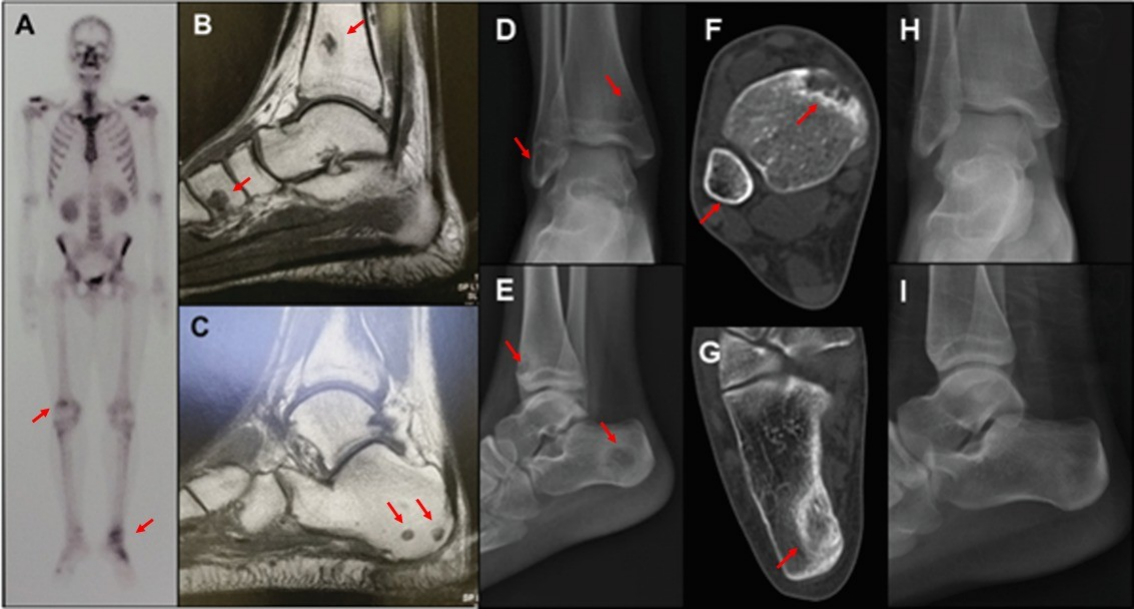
Different imaging diagnosis in bone sporotrichosis. A-C (case 40): A) Bone scintigraphy screening demonstrating uptake of the radiopharmaceutical in the right knee, left ankle, and foot. B-C) Magnetic resonance of the left ankle and foot—Round and well-defined lithic lesions in the tibia, calcaneus, and cuneiform. (Source: Laboratory of Clinical Research in Infectious Dermatology). D-I (case 22): D-E) Radiographs—Lytic lesions in the tibia, fibula, and calcaneus. F-G) Same lesions seen on computed tomography. H-I) Radiographs 11 months later, showing resolution of the lesions. The lesions are pointed by the red arrows. (Source: Service of Image of the INI).
